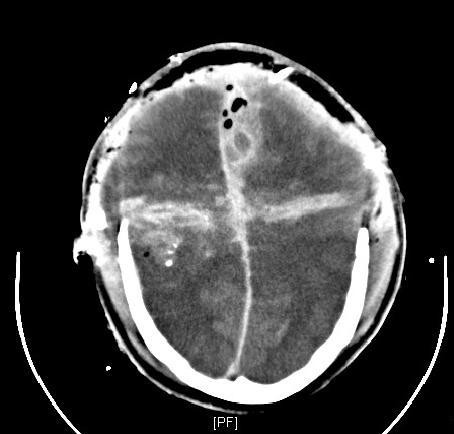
CT-scan of a lethal gunshot injury of the brain with massive swelling and increased pressure despite of craniotomie. The patient died despite of immediate craniotomie.
